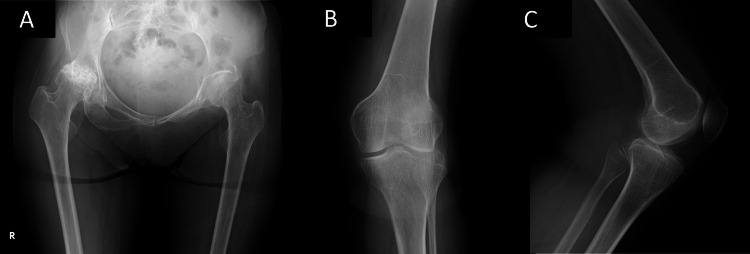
Preoperative radiographs Preoperative radiographs of the affected regions including the right hip and left knee joint. (A) Anteroposterior radiograph at the hip. (B) Anteroposterior radiographs of the knee C) lateral radiograph of the knee.
